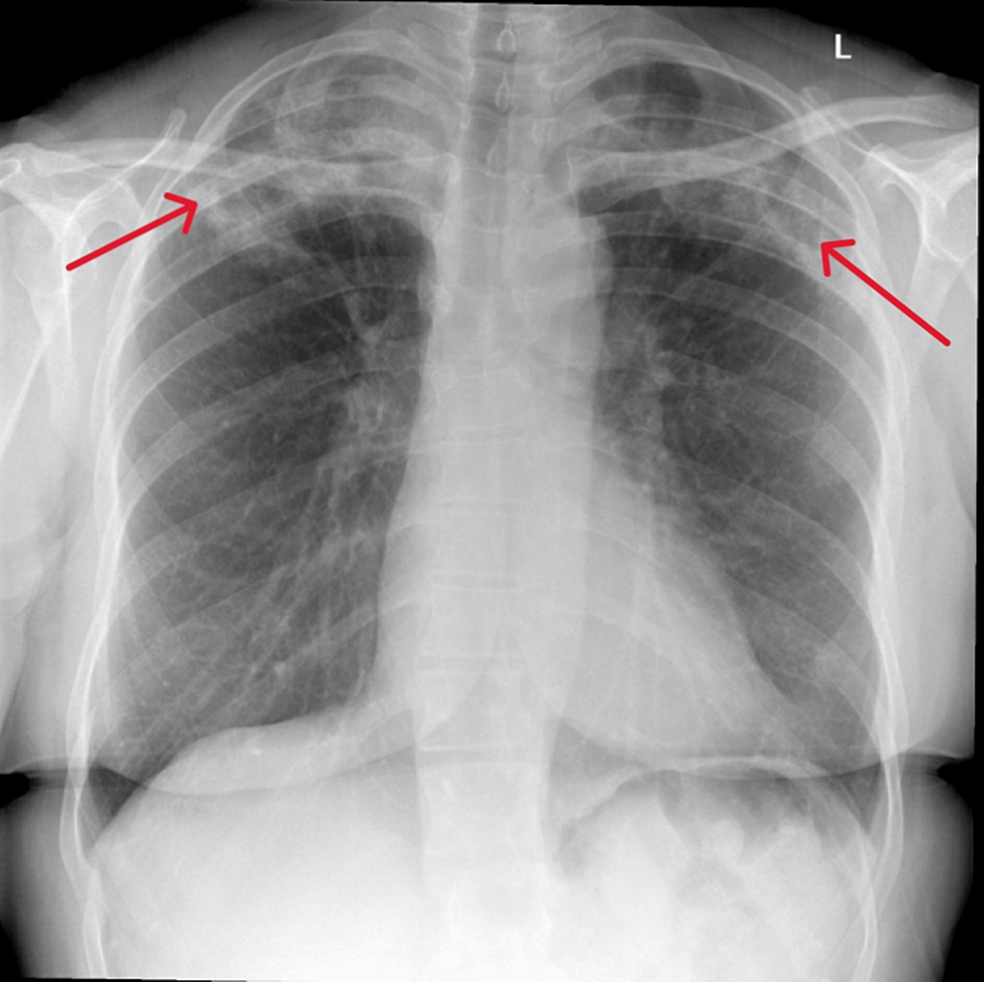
Chest X-ray taken on initial presentation. Arrows point to bilateral upper lobe opacification.
